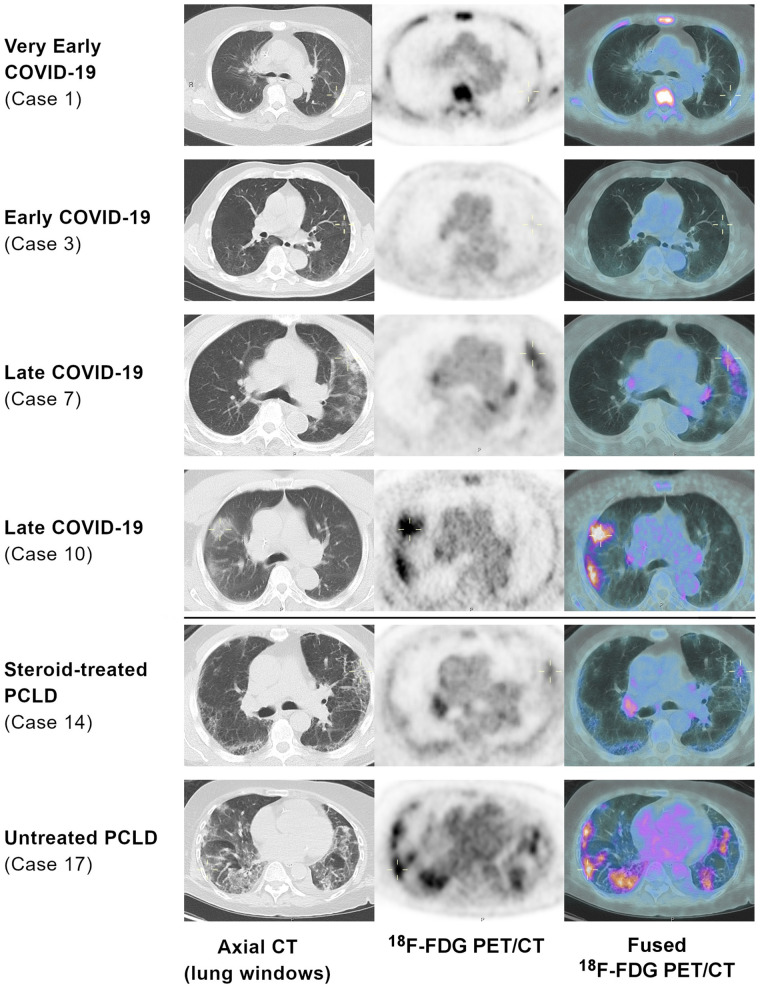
Exemplar images demonstrating increasing ^18^F-FDG uptake with temporal stage and lower ^18^F-FDG uptake in steroid-treated PCLD (lung-windowed axial CT, ^18^F-FDG PET [SUV 0–5], and ^18^F-FDG PET/CT images). Medullary uptake in case 1 was due to leukemia and not COVID-19.
